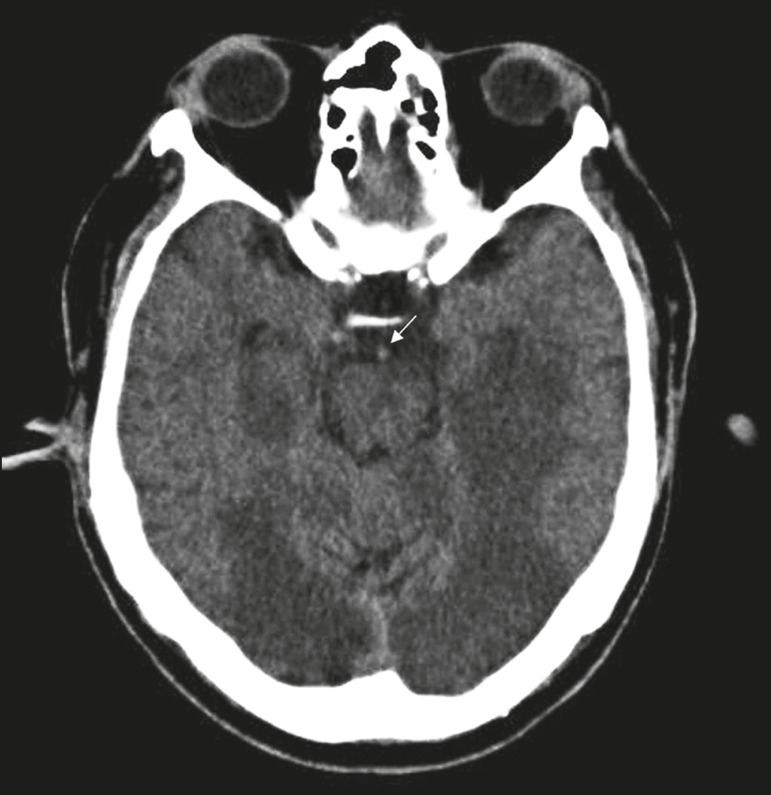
A 67-year-old male patient who presented with sudden-onset headache, blurred vision, and a reduced level of consciousness. Axial CT scan showing hypoattenuation of the cortical and subcortical regions, involving the medial temporal and occipital lobes, as well as the mesencephalon and cerebellum. Note the hyperdense artery sign, consistent with acute thrombosis, in the basilar artery (arrow).
